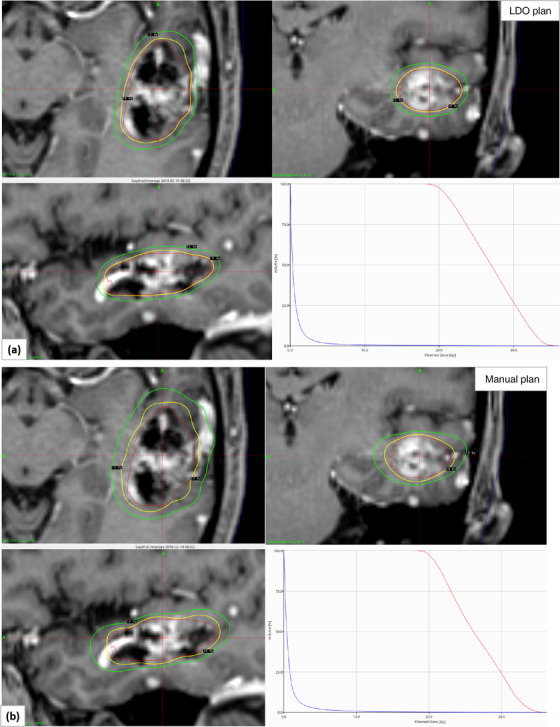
Demonstration of the axial, coronal, and sagittal views of isodose distribution (yellow, 18‐Gy prescription and green, 12‐Gy isodose lines) in the post‐contrast MPRAGE MRI images showing the difference between the lightning dose optimizer (LDO) (a, upper panel) and manual clinical plan (b, lower panel) of the representative case with the major improvement with the new inverse planning optimizer. The graphs show the dose–volume histogram (DVH) plot for nidus (red) and body contour (blue). LDO improved conformity and dose gradient and significantly reduced normal brain *V*
